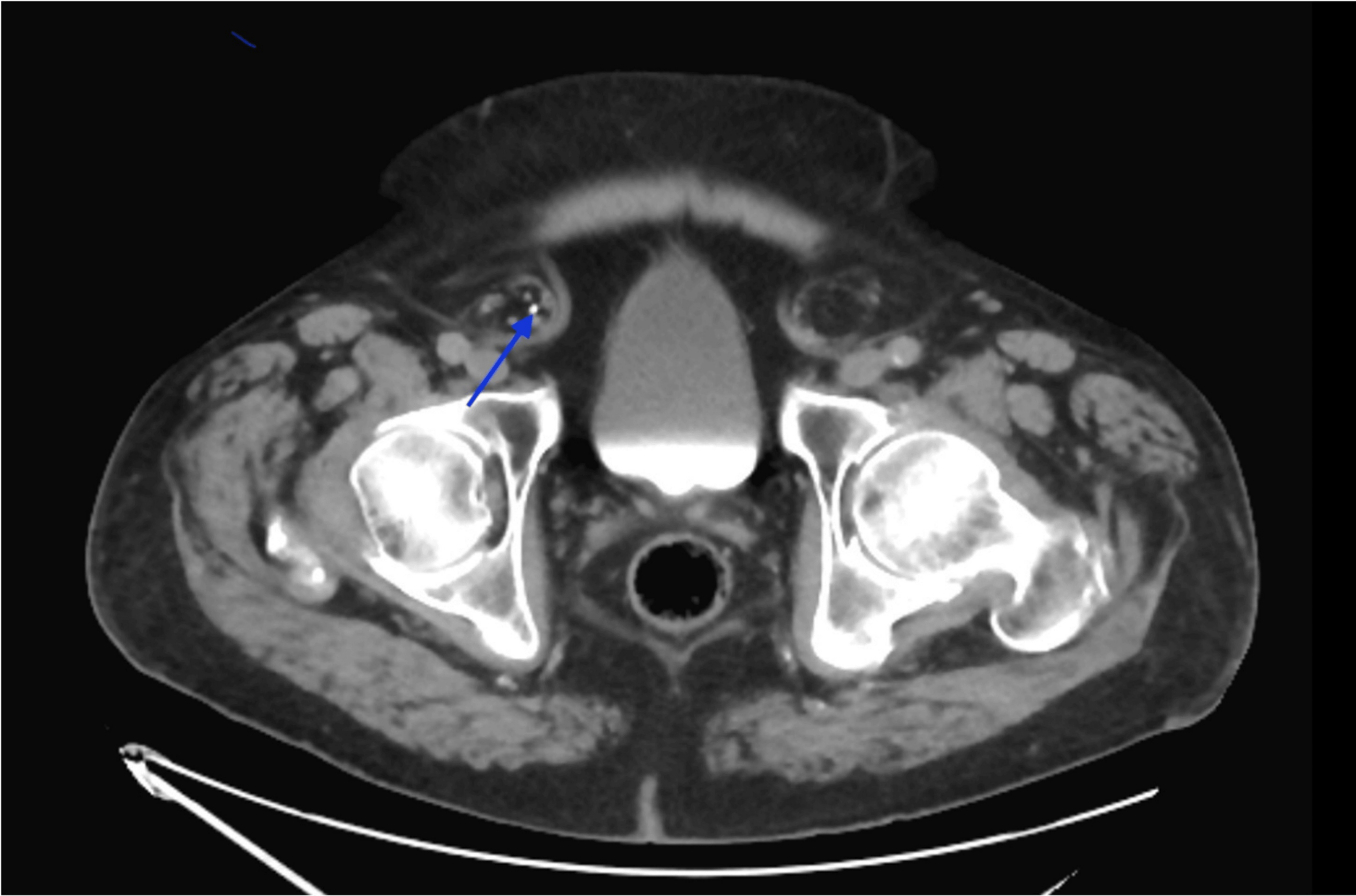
CT with contrast showing ureteral presence (blue arrow) within the inguinal hernia on the right. Still imaging made it difficult to properly display the left sided ureter.
